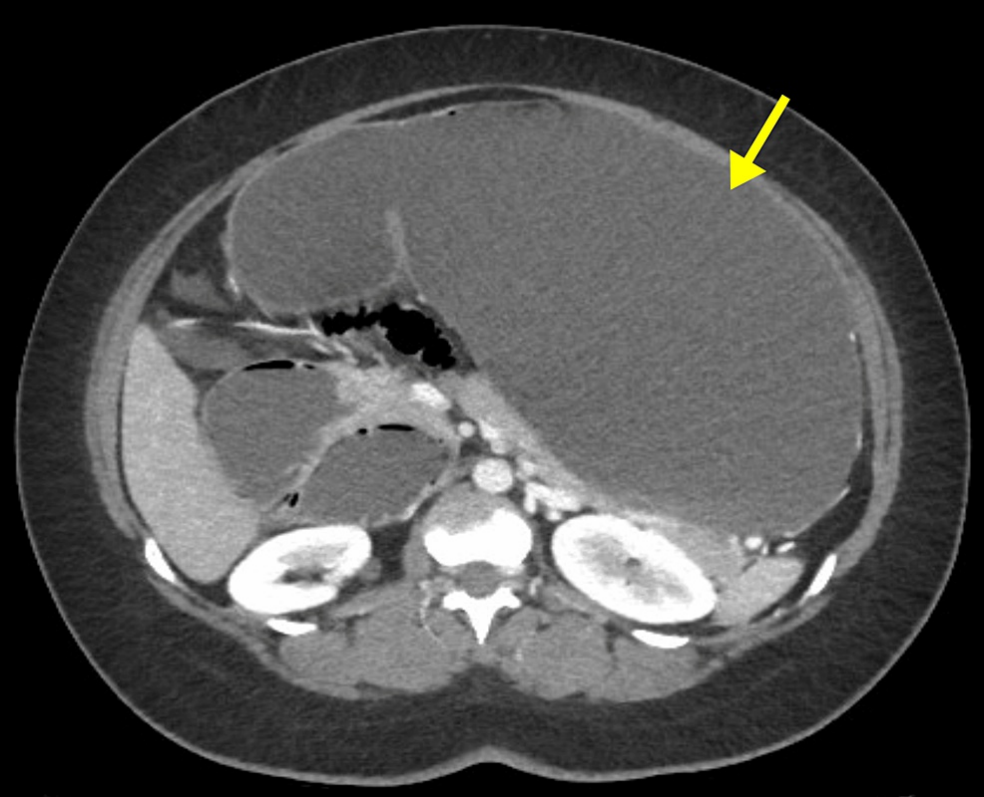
Abdominal CT with contrast shows a markedly distended stomach
